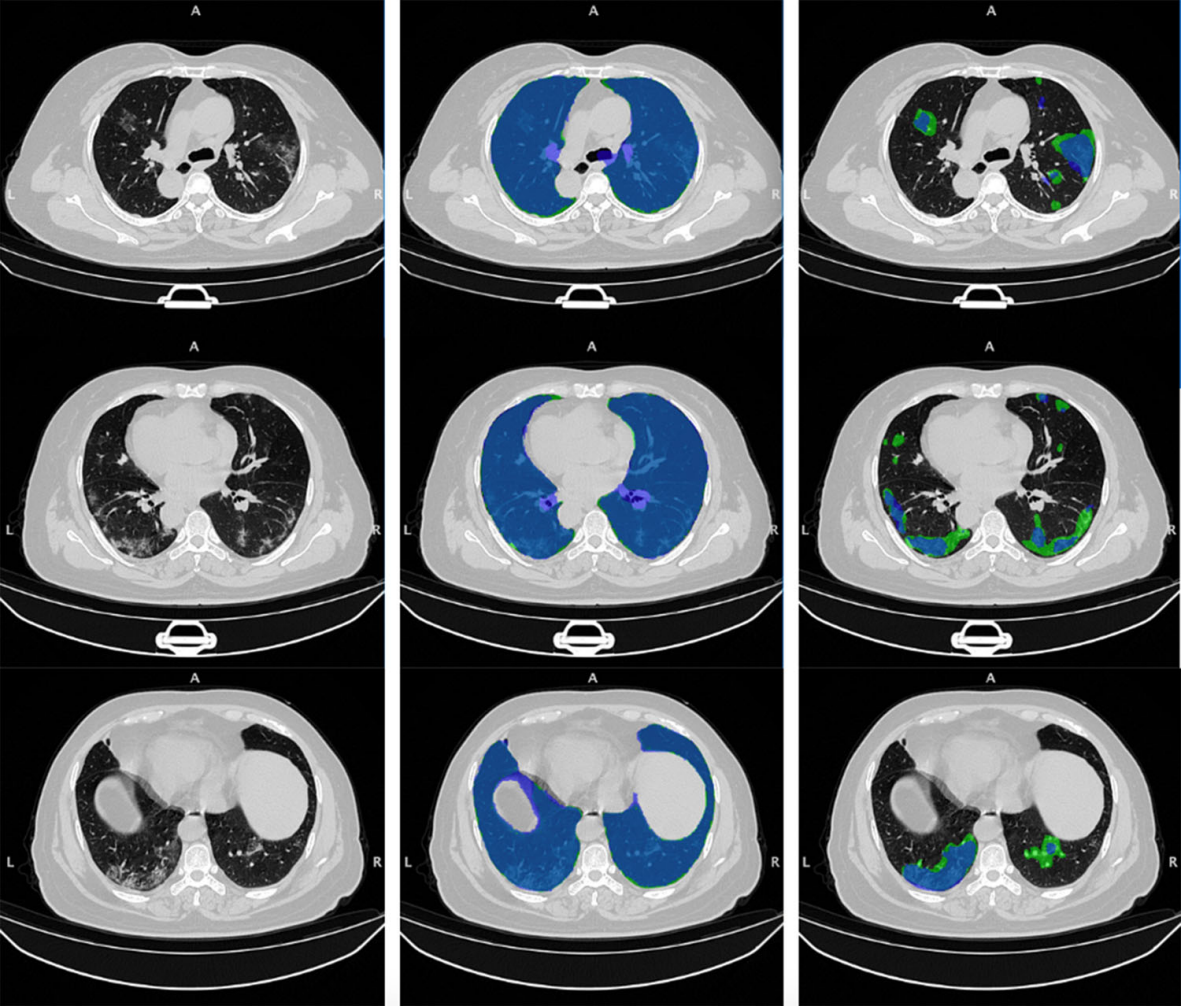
On the rows: three axial slices of the first CT scan on the COVID-19-CT-Seg test dataset (*coronacases*001.*nii*) are shown. On the columns: original images (left); overlays between the predicted and the reference lung (centre) and COVID-19 lesion (right) masks. The reference masks are in green, while the predicted ones, obtained by the *LungQuant* system integrating U-net$$_2^{90\%}$$,are in blue
